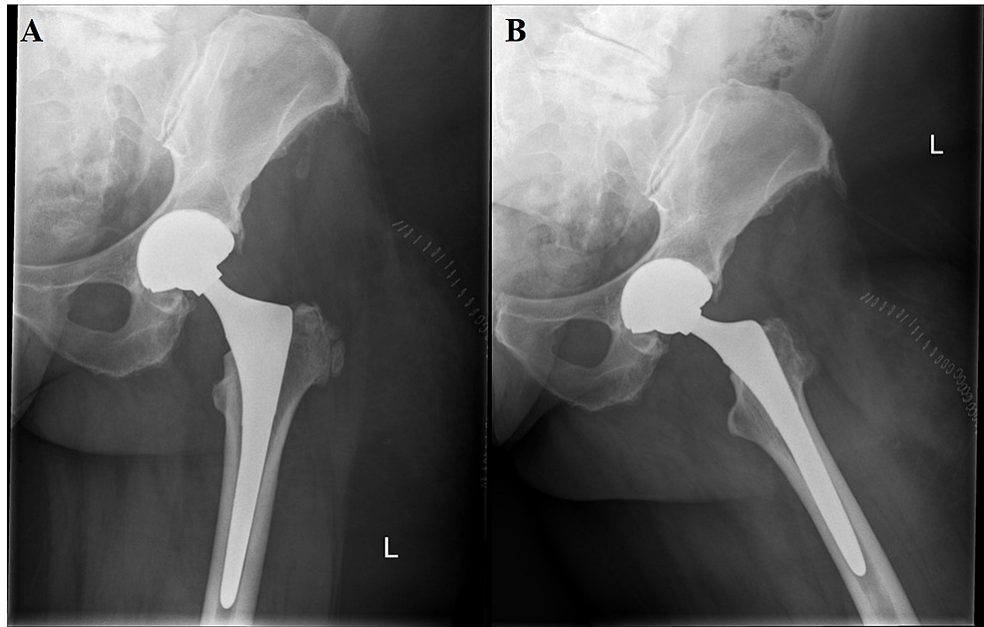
Two-week postoperative anterior-posterior (A) and lateral (B) radiographs after first revision surgery to a larger head and cup with decreased anteversion.
